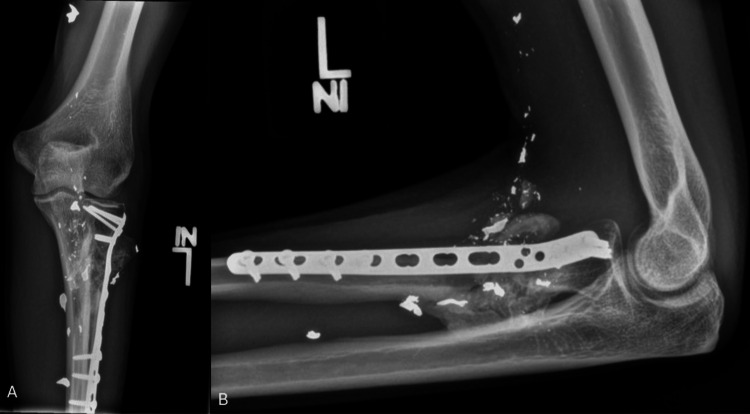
Follow-up radiographs Anteroposterior (A) and lateral (B) radiographs demonstrating a healed proximal radial shaft fracture at 18 months postop fixed with a right-sided posterolateral distal fibula plate.
